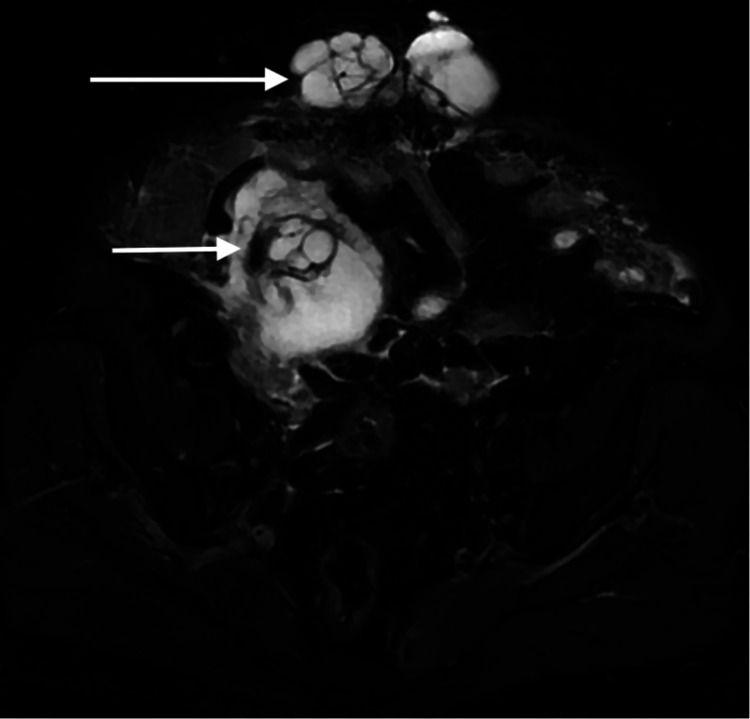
MRI of the abdomen and pelvis in an axial T2 fat sat sequence. On fat-suppressed T2WI MR of the lower abdomen, the lesion is of high signal intensity and shows multiple internal thin and smooth septations (short arrow). The mass is surrounded by high signal intensity fluid. The extension of the multi-septate lesion through the abdominal wall defect is also demonstrated in this image (long arrow).
